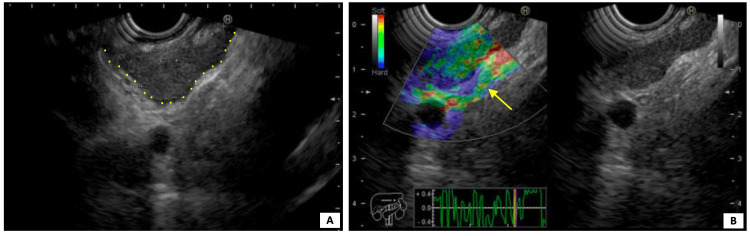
Endoscopic ultrasound-guided elastography of the larger mediastinal lymphadenopathy with 23 x 12 mm (A, yellow dots) showing a predominantly green pattern in the elastographic evaluation (B, yellow arrow), more in favor of a benign etiology
